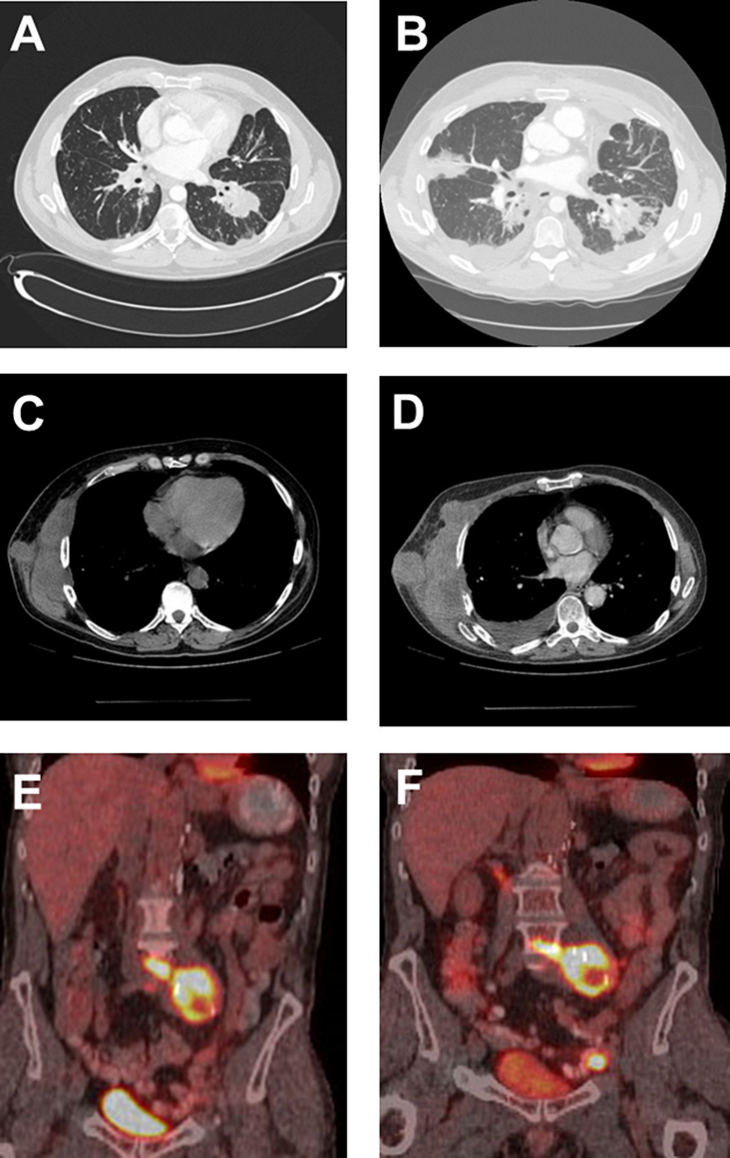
Radiographic imaging of baseline and progression of masses in RCC patients CT of the chest of patient 3 demonstrating lung nodules at baseline (A) and progression of the disease with new lesions, lymphangitic spread and worsened left pleural effusion (B). CT of the chest of patient 7 demonstrating right chest wall mass at baseline (C) and subsequent progression of disease with increase in size of this target lesion (D). A PET-CT fused coronal image of patient 4 showing intensely FDG-avid retroperitoneal lymph nodal mass (SUV of 12.1) at baseline (E) and subsequent development of new left external iliac lymph node (SUV of 22) as well as mild increase in the FDG activity of the retroperitoneal lymph nodal mass (SUV of 14.8) (F).
